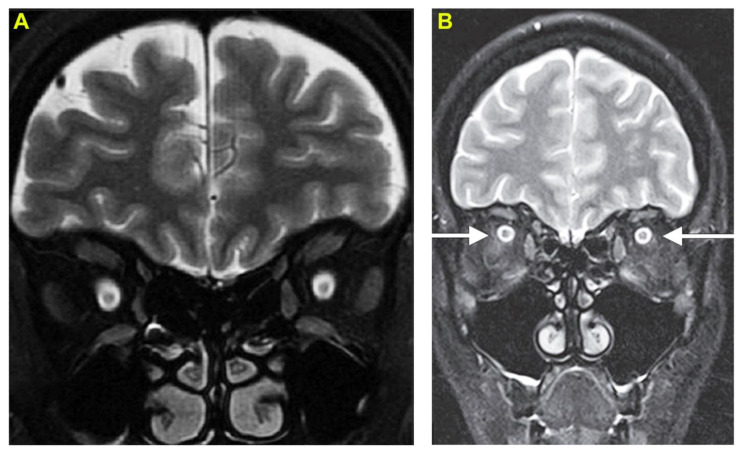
Optic neuritis following envenomation by a common krait. (A) coronal T2-weighted FLAIR magnetic resonance imaging shows high signal changes in both the optic nerves. (B) coronal T2-weighted magnetic resonance imaging with fat suppression indicates enlarged optic nerves surrounded by cerebrospinal fluid providing a ‘polo-mint’ appearance (indicated by arrows).
